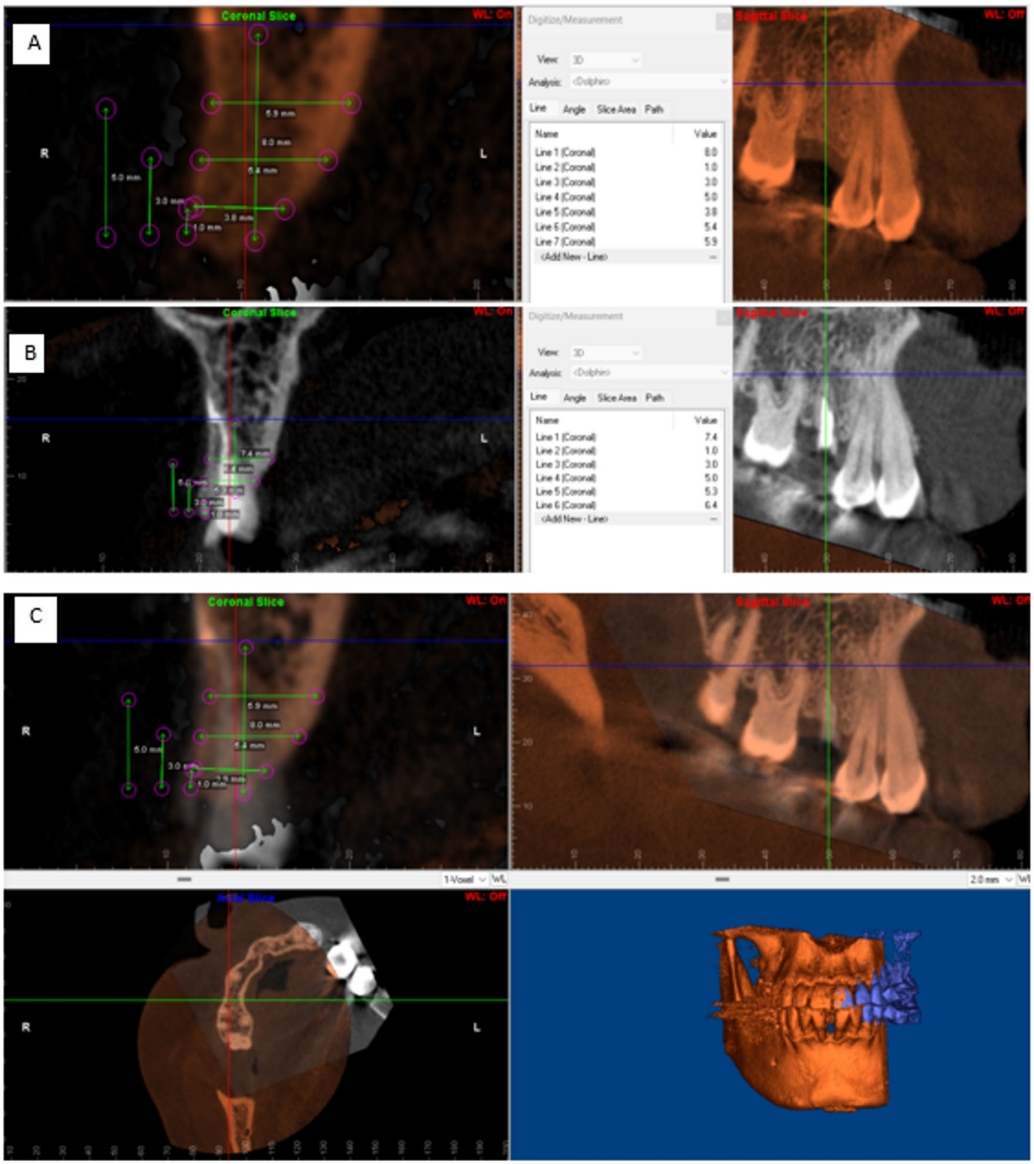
Photographs of bone measurements (height and width) on CBCT cuts showing (A); preoperative CBCT for non-restorable upper right second premolar (B); measurements after extraction and (C) photographs of axial, sagittal, and coronal CBCT cuts showing fusion between primary and secondary images.
